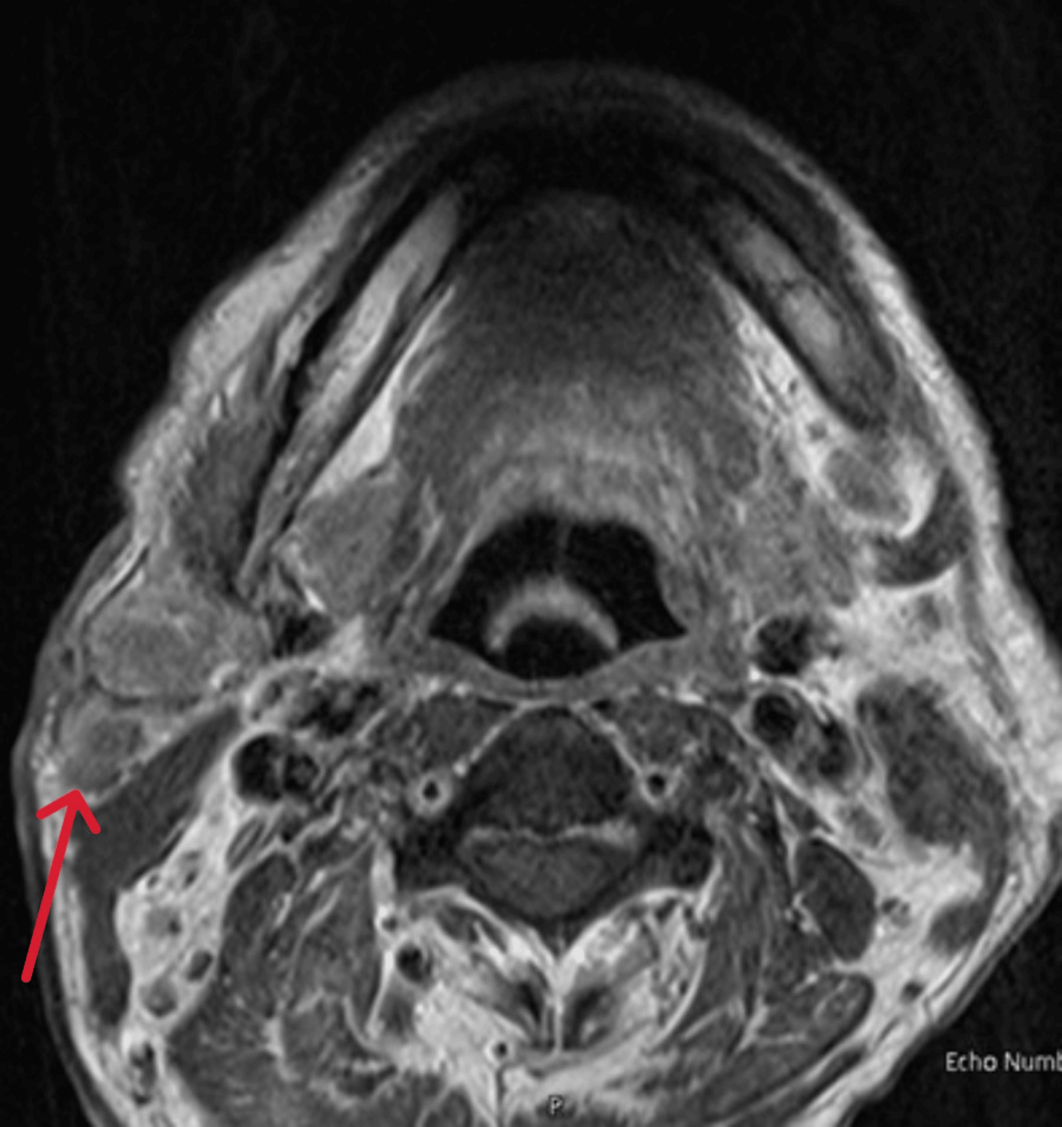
MRI of the face revealed central cystic necrosis right parotid gland (red arrow).
